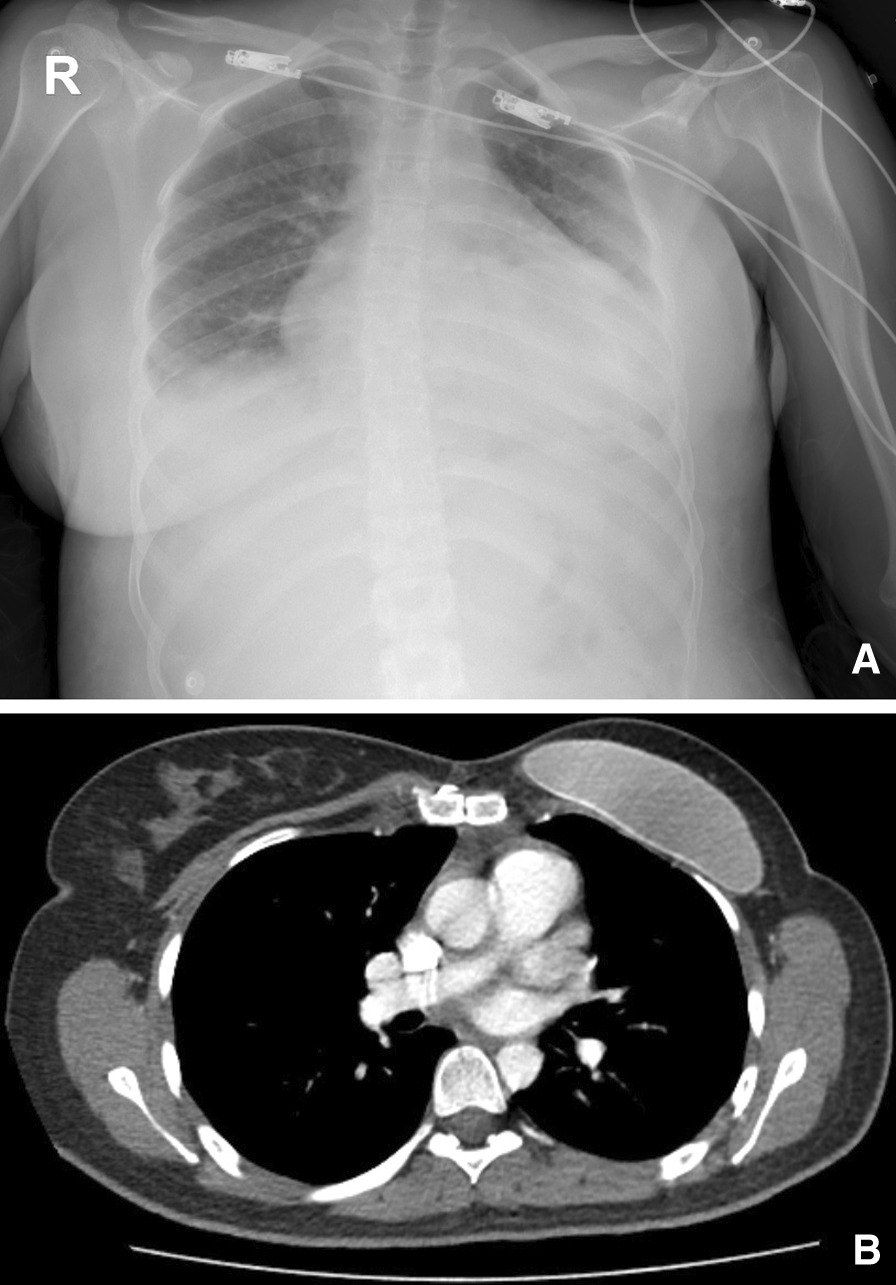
A Preoperative chest X-Ray showing absence of left breast tissue. B Postoperative CT- angiography showing absence of left pectoralis muscle and left artificial breast
